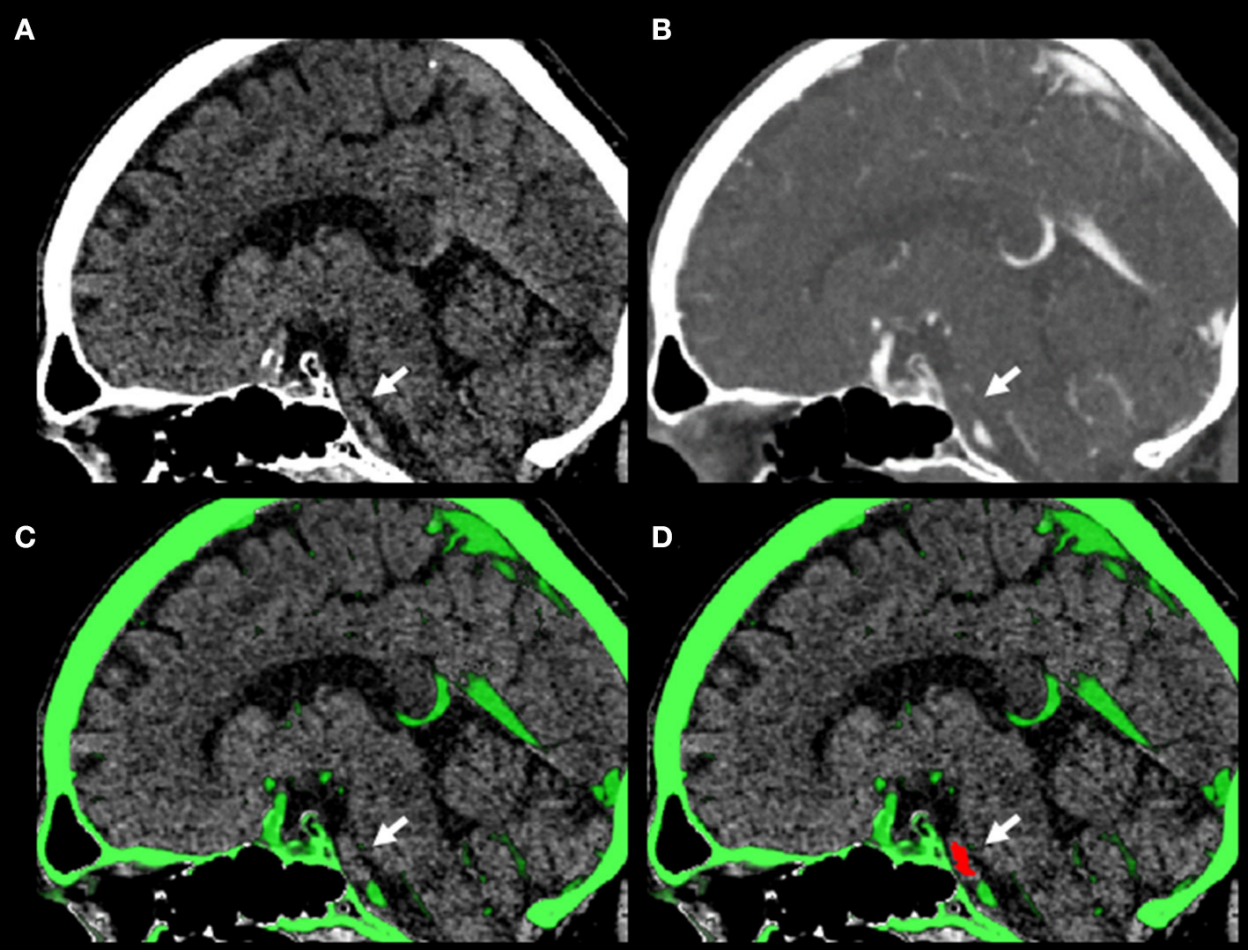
Example of a 66-year-old male patient presenting with a National Institutes of Health Stroke Scale (NIHSS) score of 20. No intravenous thrombolysis was performed due to arrival beyond 4.5 h since symptom onset. Mean thrombus attenuation increase (TAI) was 9.11 Hounsfield units, and clinically determined most likely stroke etiology was large artery atherosclerotic (LAA). Endovascular therapy was performed using a Sofia 6F (Plus) device. The patient was discharged with an NIHSS score of 10 and a 3-month follow-up modified Rankin Scale (mRS) score of 4. (A) Non-contrast computed tomography (NCCT). (B) CT angiography (CTA). (C) Overlay of NCCT and CTA. (D) Overlay of NCCT and CTA with segmentation of the thrombus in the basilar artery. Arrows indicate location of the thrombus. Shown are sagittal slices due to better visualization of the extent of the thrombus; actual thrombus segmentation was performed on axial slices.
